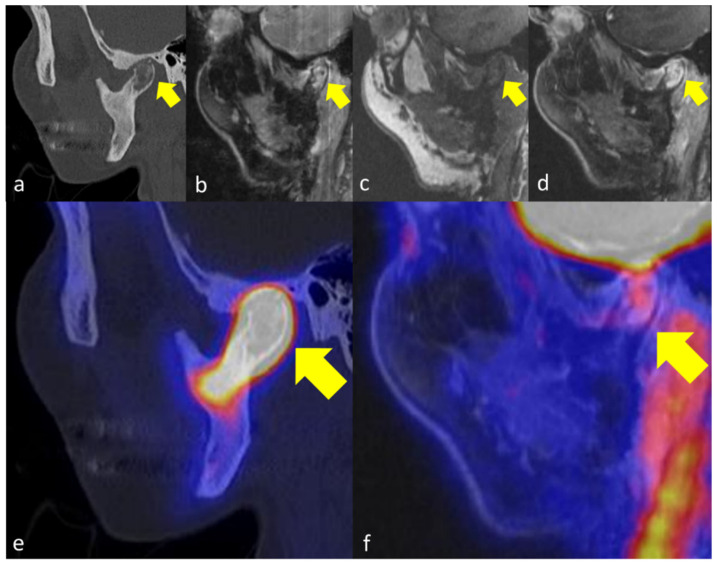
49 years old female patient with primary chronic OMJ in the right mandibular condyle (yellow arrows). CT (a) reveals diffuse trabecular sclerosis and cortical erosion. In the erosive area of the bone, a corresponding edema (b,c) can be observed as well as increased gadolinium enhancement (d). [18F]fluoride-PET/CT (e) and [18F]FDG-PET/MRI (f) reveal markedly increased tracer uptake in the OM affected jawbone below the extent of sclerosis.
